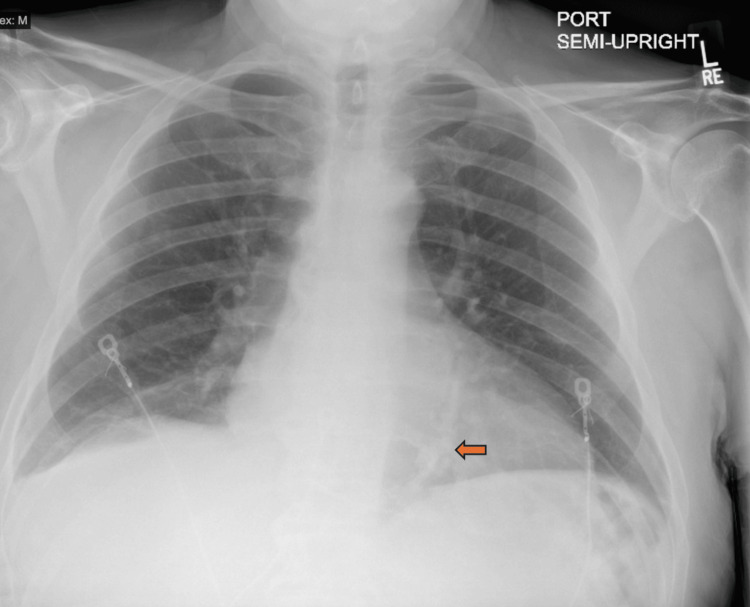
Chest X-ray showing curvilinear pericardial calcifications (arrow).
